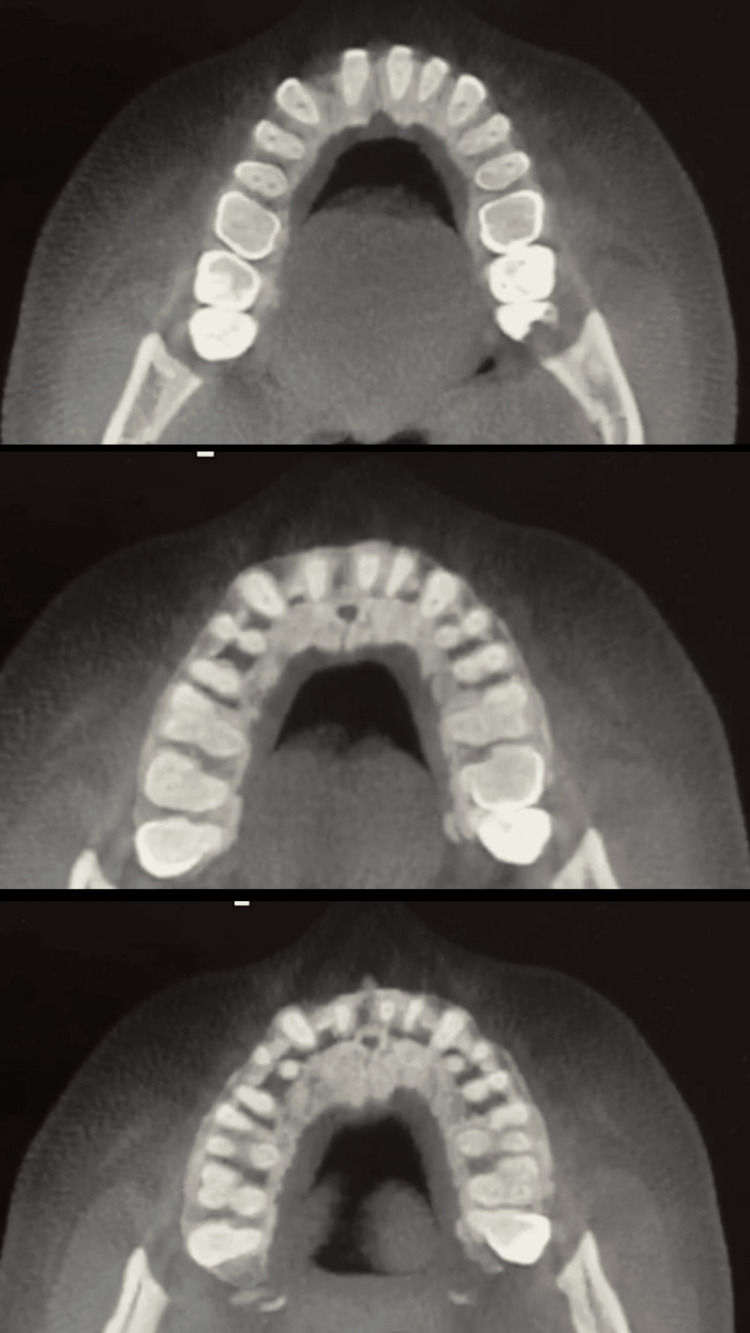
CBCT images show the bone density CBCT, cone-beam computed tomography
